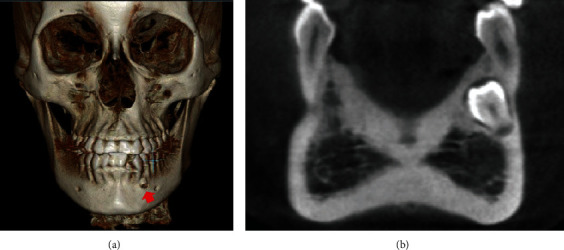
(a) 3D frontal view of the CBCT where the supernumerary tooth is visualized (red arrow) and (b) Coronal section of the supernumerary tooth showing no evidence of contact with the first lower premolar.
